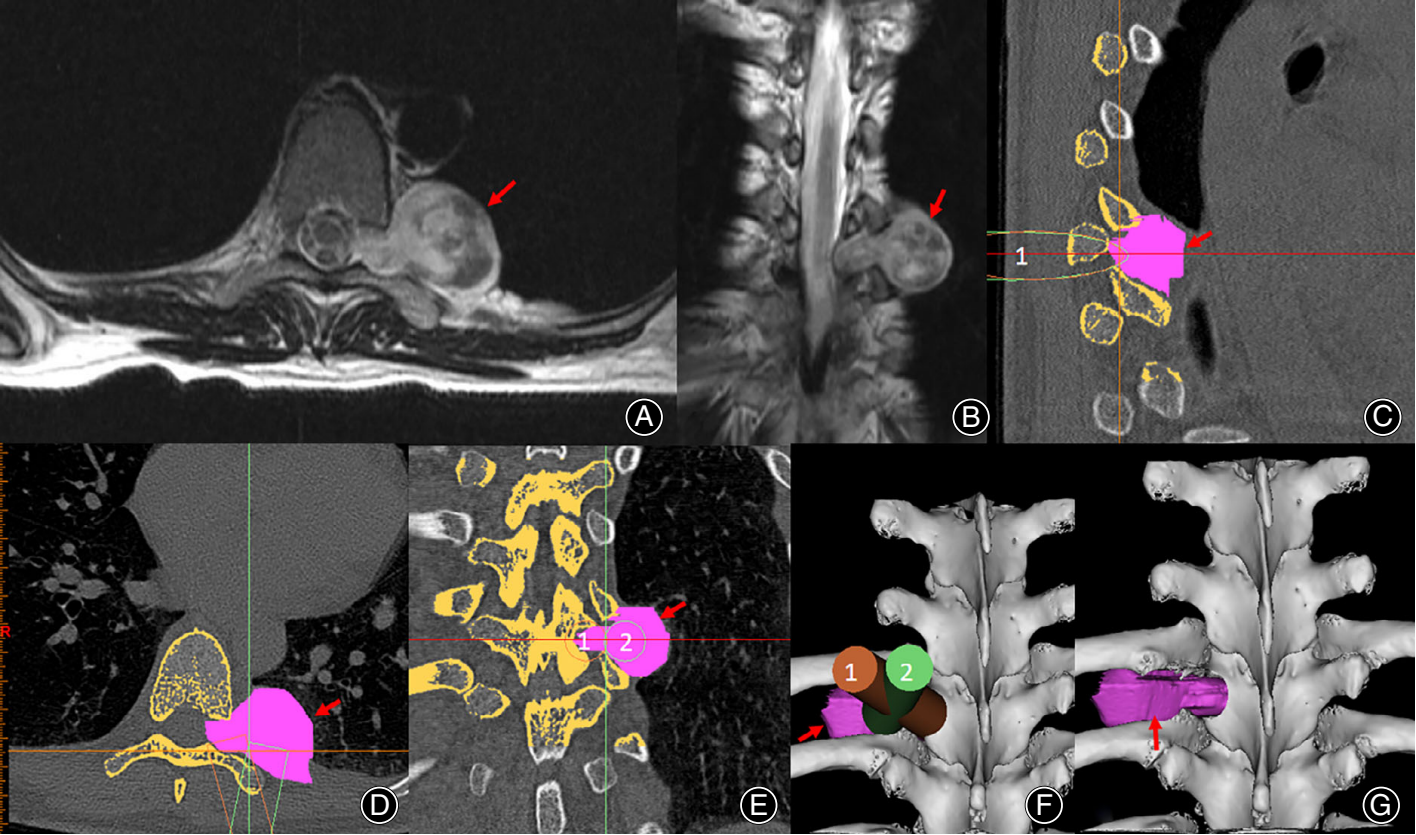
Preoperative T2‐weighted MRI on axial (A) and coronal (B) planes, revealing a dumbbell tumor in the left T8–9 foramen (arrows). Rebuilding the relationship between the vertebrae (yellow) and the dumbbell tumor (purple). Designing the operational approach with micro‐tubule (number 1, brown and number 2, green) using 3D software. Location of the tumor after partial resection of vertebrae (G).
